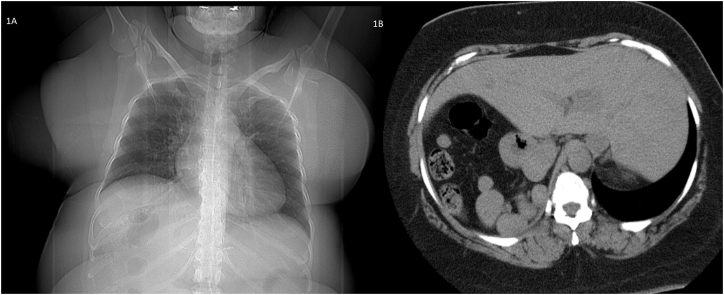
A: Chest X-ray. The heart is seen in normal position; B: Abdominal CT: Liver I noticed in the left side of the abdomen.
